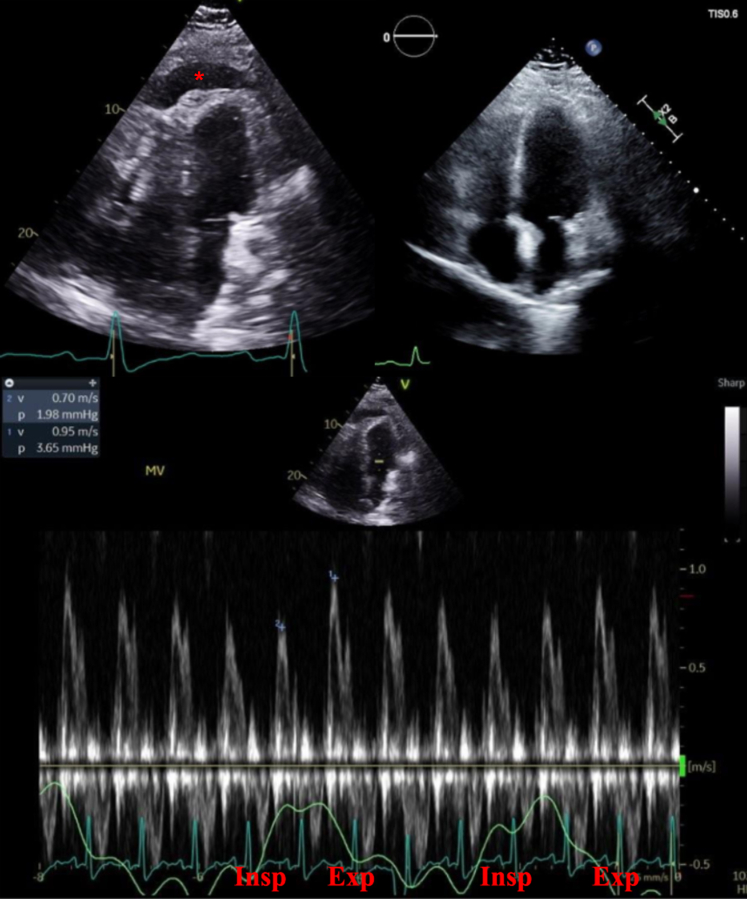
Case Presentation #1 A 50-year-old male who underwent a 4-vessel coronary artery bypass grafting which was complicated by Dressler syndrome. The upper left echo image shows a large PEff on a postoperative transthoracic echocardiogram apical 4-chamber view (red asterisk marks the PEff). The upper right echo image reveals the same TTE view with resolution of that PEff after treatment with aspirin and colchicine. The bottom image again shows the PEff on echocardiogram along with respiratory variation across the mitral valve on Doppler inflow pattern. Exp = expiration; Insp = inspiration; PEff = pericardial effusion; TTE = transthoracic echocardiogram.
